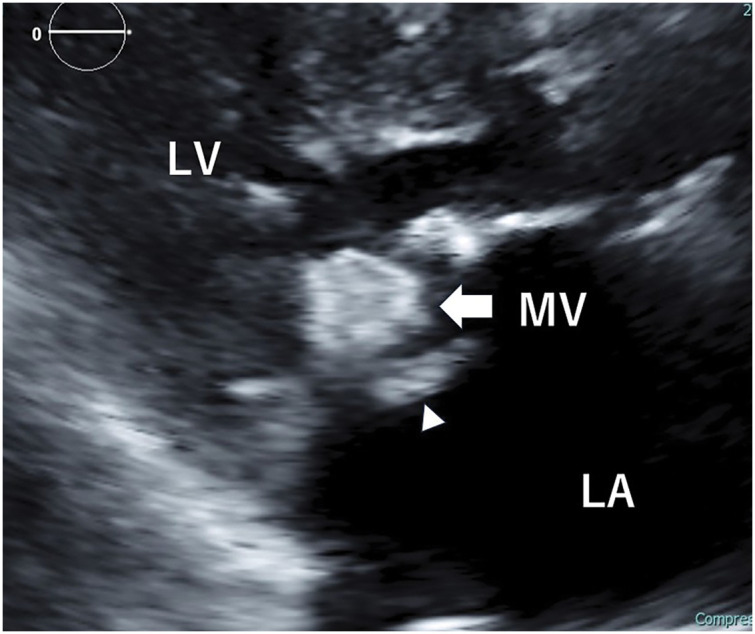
Parasternal long axis view of a transthoracic echocardiogram. The posterior mitral valve leaflet exhibits a calcified, tumor-like appearance (arrow) accompanied by mobile vegetation (arrowhead). LA, left atrium; LV, left ventricle; MV, mitral valve
